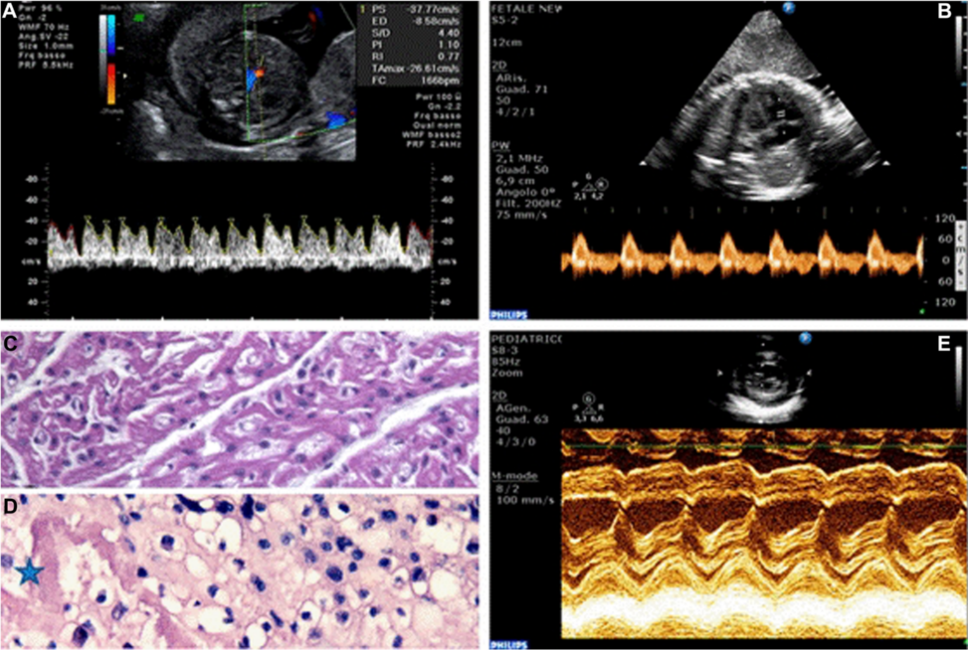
Panel a: Sonogram showing a significantly elevated DV-PIV in twin A. Panel b: Echocardiogram of twin B. Four chamber view. PW Doppler of flow through tricuspidal valve. Monofasic diastolic filling of the right ventricle; hallmark of diastolic function. Panel c: Myocardial severe interstitial edema with fiber dissociation and sporadic vacuolar myocyte degeneration of the twin A fetal heart. Panel d: Hypertrophic vacuolization and nuclear pleomorphism of extravillous throphoblast, with interstitial edema and areas of fibrinoid necrosis of placenta (✷). Panel e: Neonatal echocardiography of twin B. M-mode long axis of the left ventricle. Evidence of mild septal hypokinesia with an overall preserved global contractility
